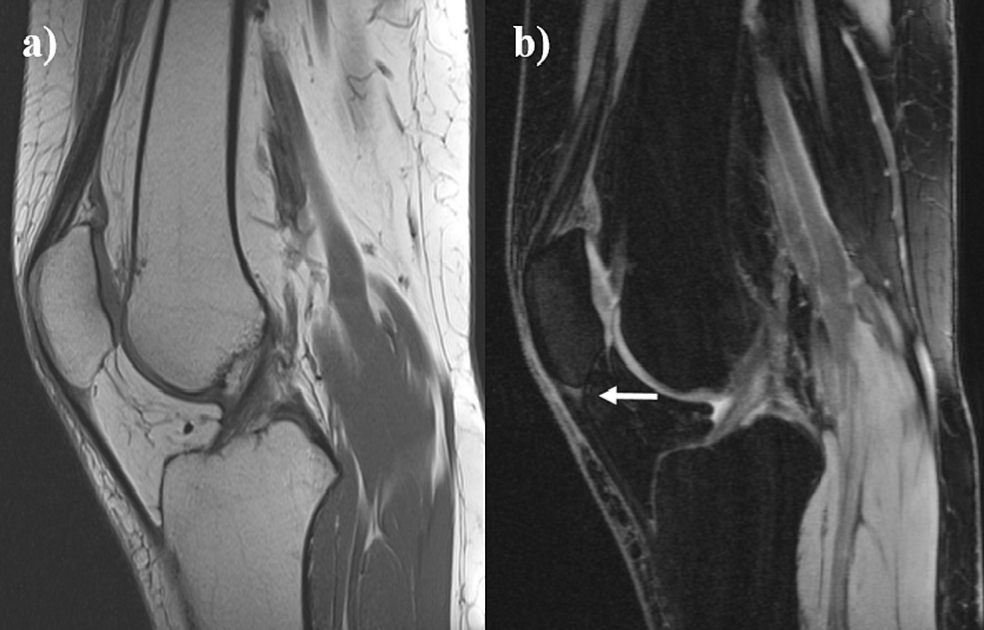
MRI images of the patient diagnosed with patellar tendinitis a) T1-weighted image b) T2-weighted image shows increased signal intensity at the inferior pole of patella (arrow).
